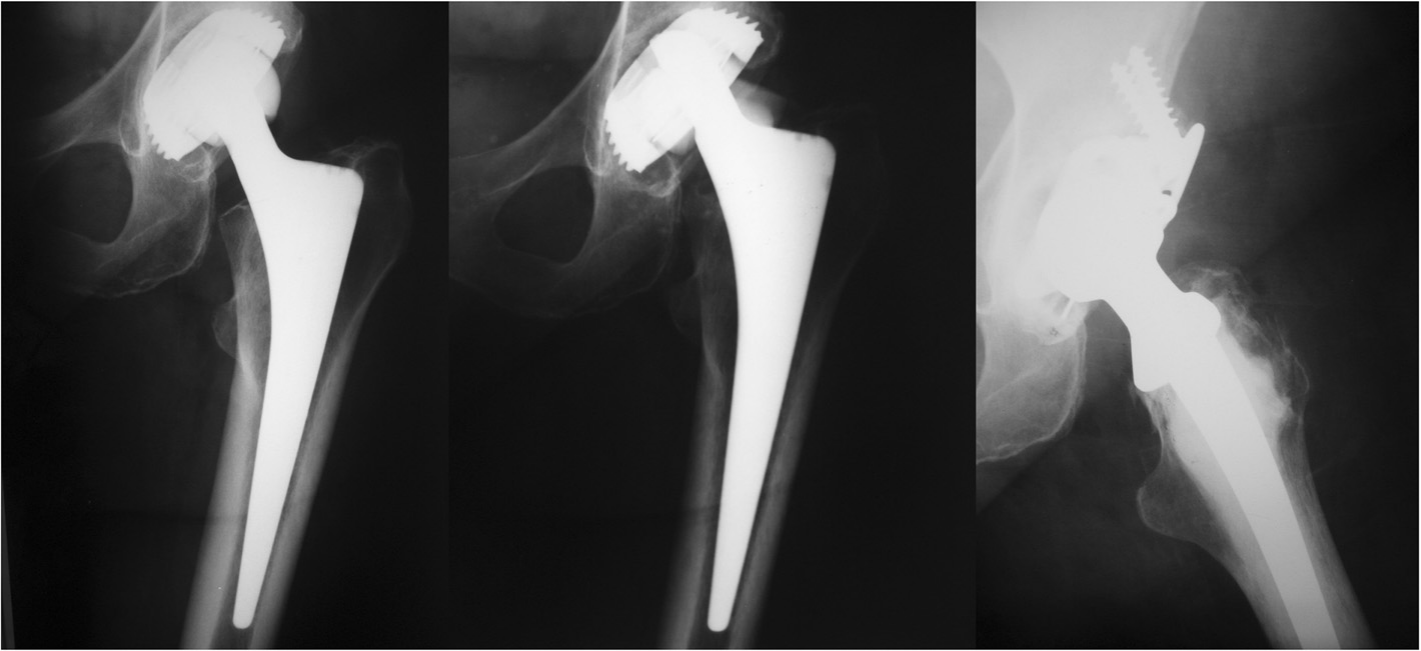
Radiographs of the hip with the fractured ceramic head. Left: directly post-OP: Middle: Fracture of ceramic head at 6.25 years postop; Right: After revision arthroplasty
